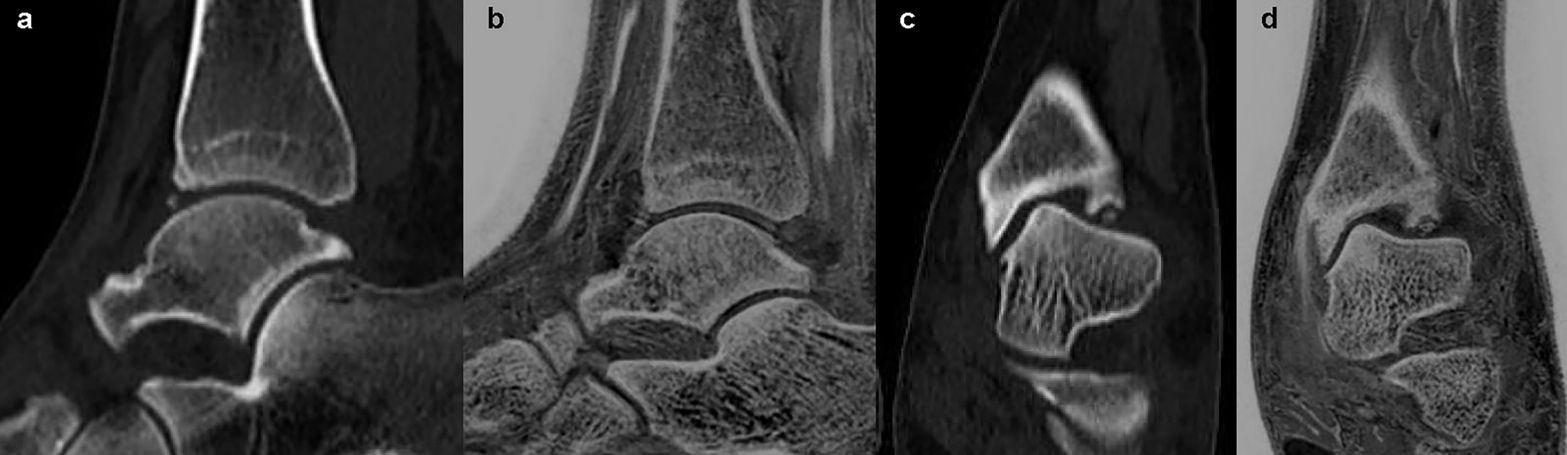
Images in a 36-year-old female with anterior and inferior avulsion fracture of left tibia. (A, C) were sagittal and coronal CT images. (B, D) were sagittal and coronal FRACTURE images. Free bone fragments were consistent in CT and FRACTURE images.
